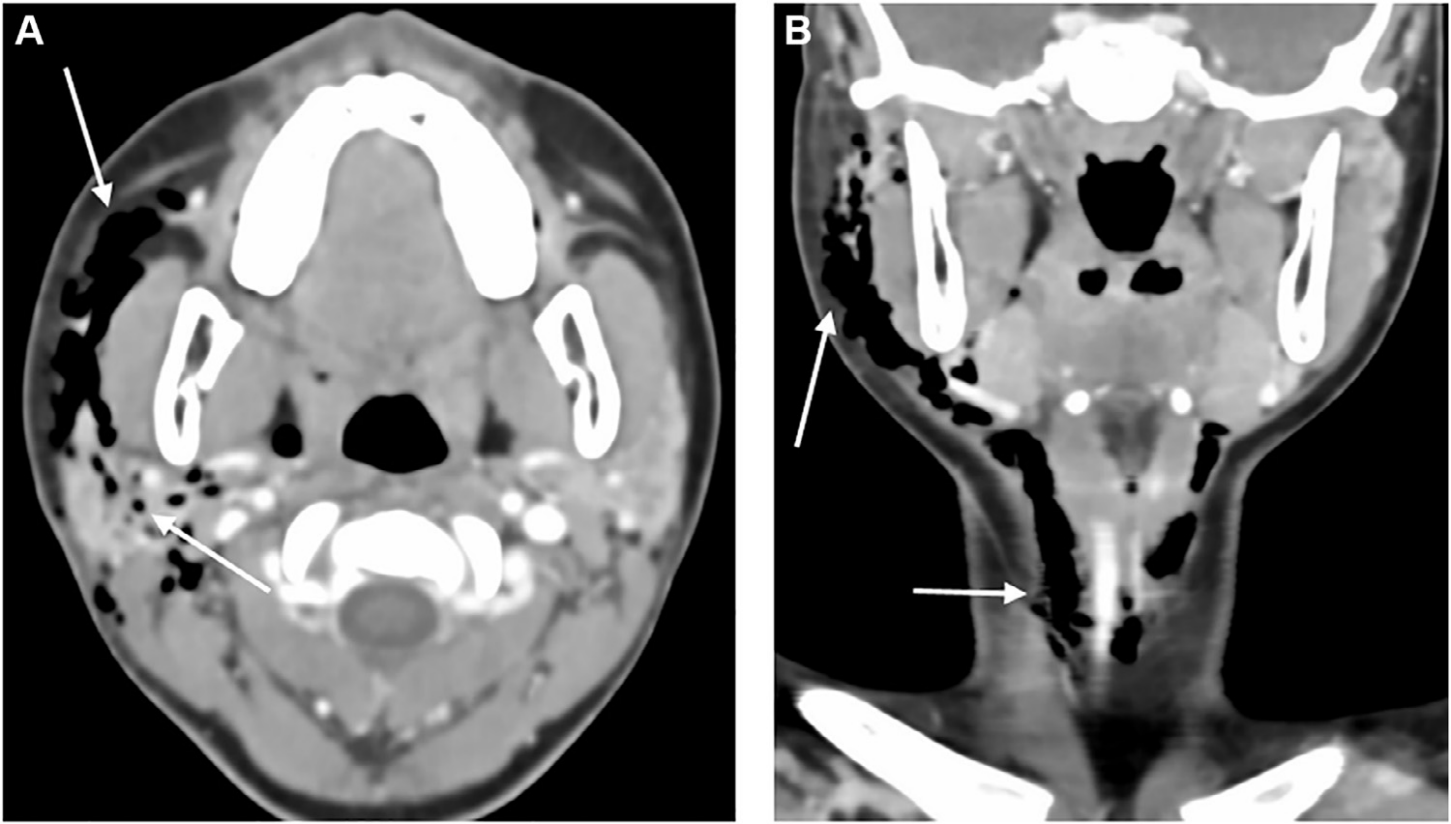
A axial and coronal CT showing recurrence of the pneumoparotid and cervical emphysema, on the right side.
